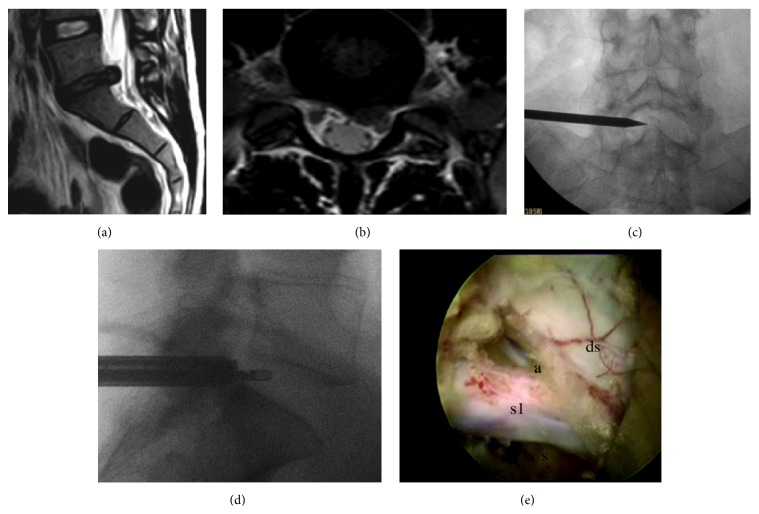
(a-b) Preoperative T2-weighted sagittal and axial MRI showing an L5-S1 disc herniation impinging the left S1 nerve root. (c-d) Intraoperative fluoroscopy showing different phases of the transforaminal approach. AP view: pointer showing the L5-S1 interlaminar window and lateral view showing the radiofrequency bipolar endoscopic probe inside the L5-S1 intervertebral disc. (e) Removal of the herniated disc material has been completed. At the end of the procedure, the dural sac (ds), the S1 nerve root (s1) with its axilla (a), and shoulder (s) can be clearly visualized. Taken from the senior author's personal series.
